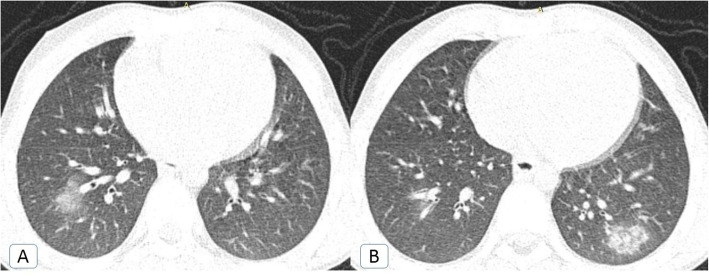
Typical COVID-19 CT signs in a 7-year-old boy: A, B Axial chest CT cuts (lung window) showing bilateral lower lobar ground glass patches with evolving left basal consolidative changes
